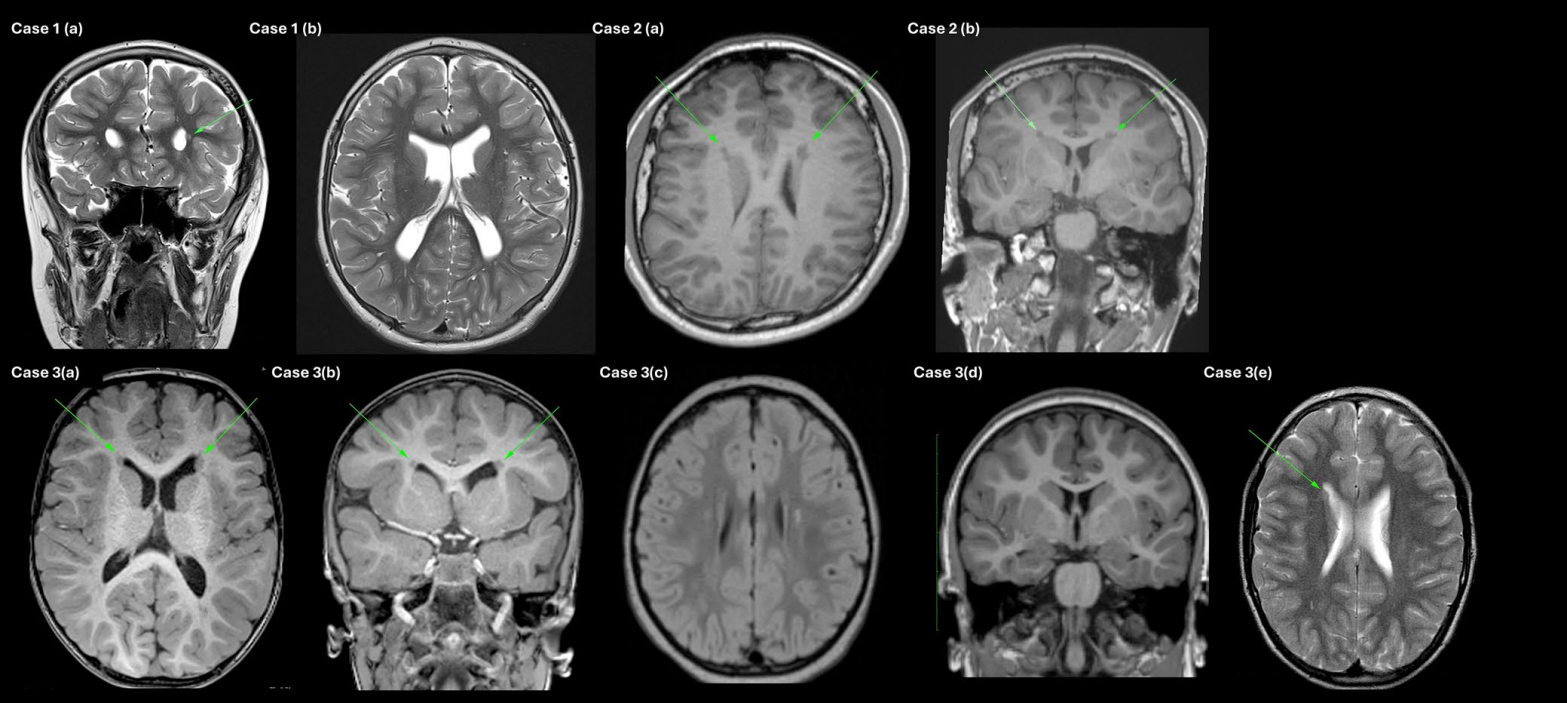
MRI brain images from patients (arrows indicate PVNH). Case 1 coronal (a) and axial (b) T2 weighted images demonstrating PVNH around the left frontal horn and prominent ventricles and perivascular spaces. Case 2 Axial (a) and coronal (b) T1 weighted images, demonstrating PVNH around the frontal horns. Case 3. Axial (a) and coronal (b) T1 weighted images of III‐5 demonstrating PVNH. Axial FLAIR (c) and coronal T1 weighted (d) images of III‐1 showing abnormal T2 high signal in the corona radiata, but no evidence of PVNH. Axial (e) T2 weighted image of the father (II‐3)'s brain demonstrating a small focus of PVNH adjacent to the right frontal horn. [Colour figure can be viewed at wileyonlinelibrary.com]
